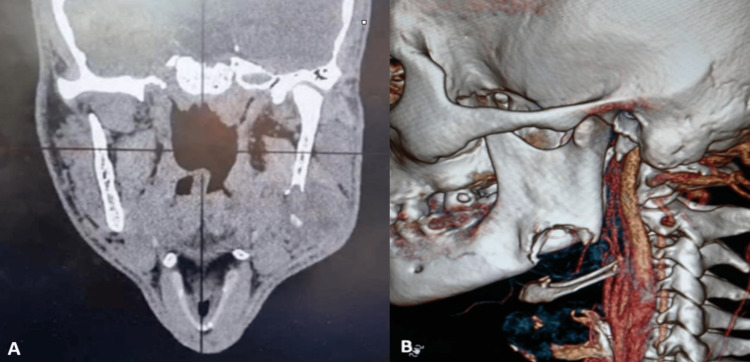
(A) A coronal computed tomography (CT) revealed a prominent 4 x 3 cm mass in the left mandibular angle area and cortical destruction was evident medially, laterally, and inferiorly, with extension into the surrounding soft tissues, (B) 3-D revealed a cortical destruction in the left mandibular angle.
